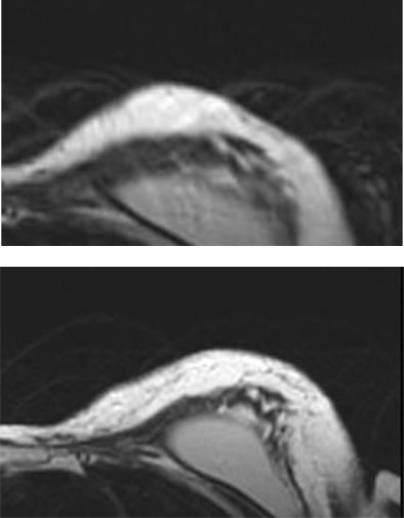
Post-operative evaluation of graft retention by MRI at 3 months
